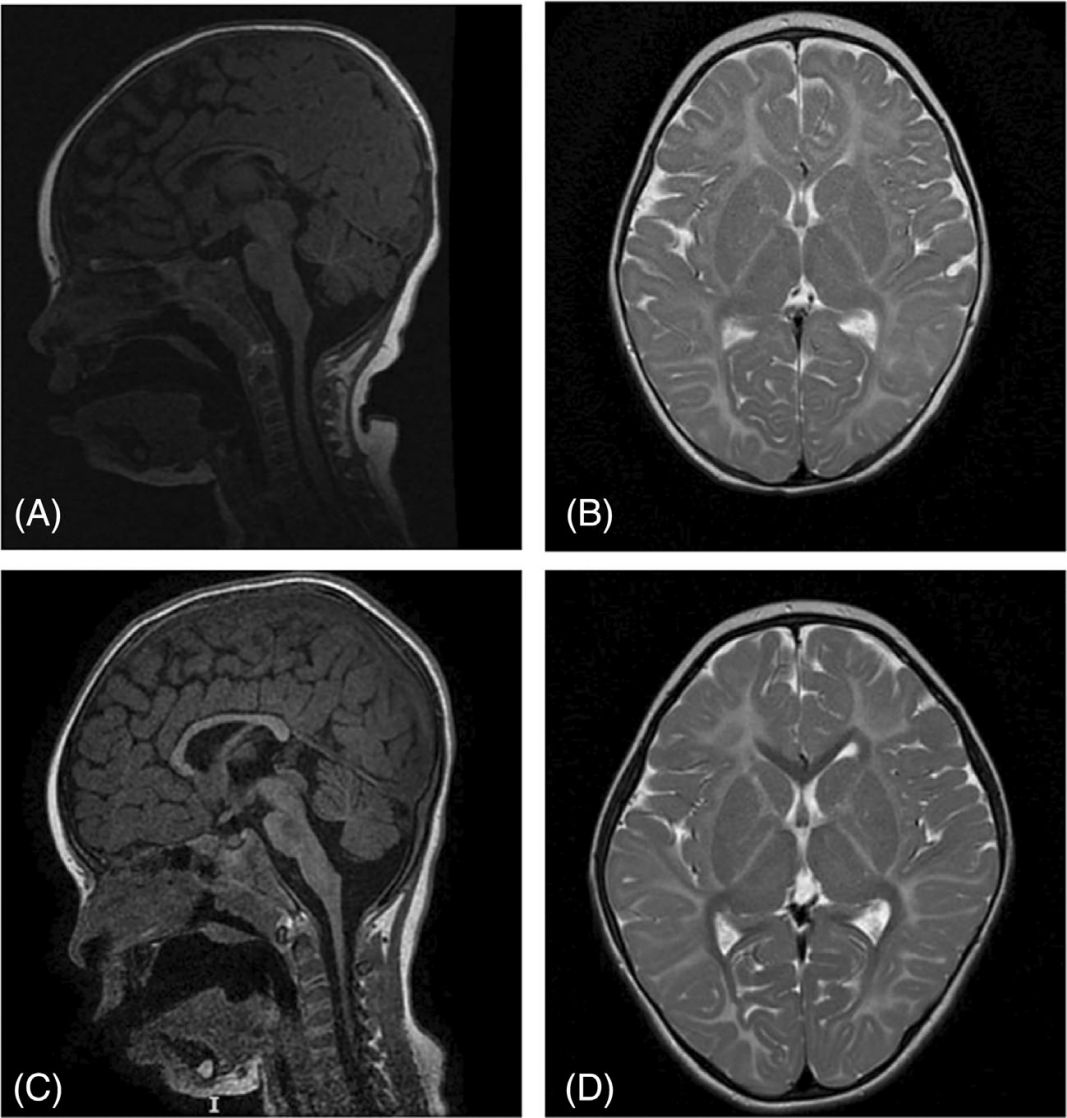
Sagittal T1 (A, C) and axial T2 (B, C) images of Patient 2 at 8 months (A, B) and 3 years (C, D) of age. Comparing the initial MRI images with the follow‐up MRI a delayed myelination for the age can be observed. By the age of 3 years white matter myelination has occurred centrally in the corpus callosum (T1‐weighted images). No significant increase of the volume of corpus callosum and cerebellum.
